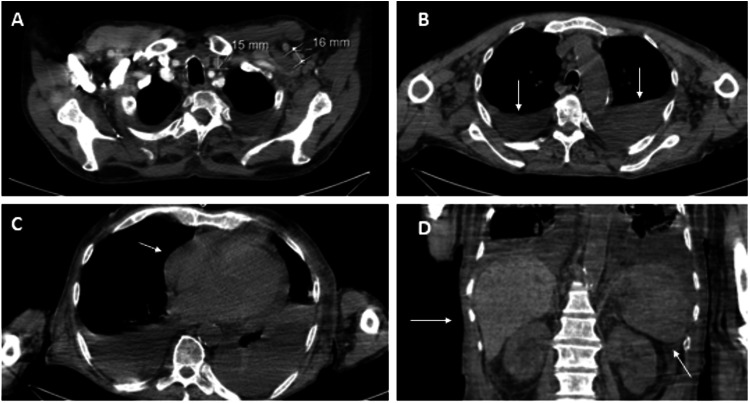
Findings on CT scan. A: Multiregional lymph nodes over 1 cm in diameter. B: Pleural effusion. C: Pericardial effusion. D: Hepatomegaly and splenomegaly.
